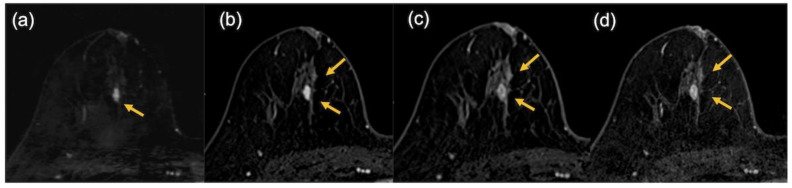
The images of a patient with luminal subtype breast cancer after neoadjuvant endocrine therapy in combination with cyclin-dependent kinases 4 and 6 inhibitor. In the pathological findings, 12 mm of invasive cancer remained. In the MRI findings, the lesion was evaluated as being slightly smaller on the ultrafast dynamic contrast-enhanced MR image (9.5 mm) (a) but larger in the early phase (21.5 mm) (b), the delayed phase (24 mm) (c), and the high spatial-resolution (24.5 mm) (d) images (arrows).
